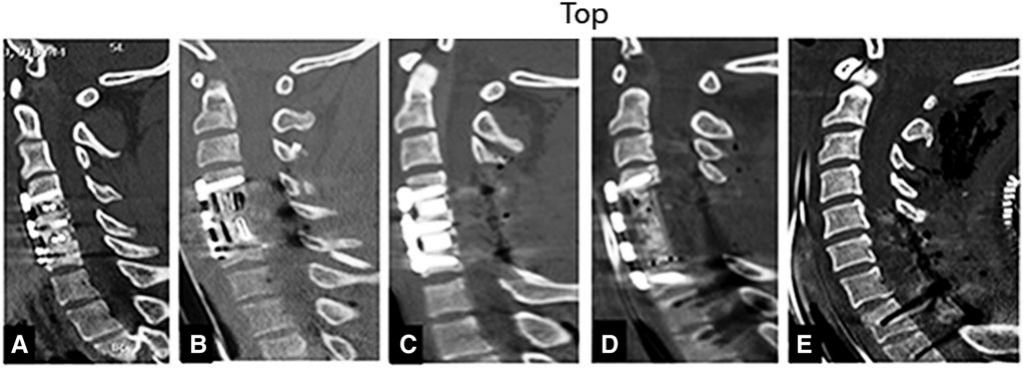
Midsagittal CT scan of cervical spine indicating (A) anterior cervical discectomy and fusion (ACDF); (B) anterior cervical corpectomy and fusion (ACCF); (C) Combined ACDF and three level laminectomy and posterior spinal fusion (PSF); (D) ACCF (two levels) and Laminectomy (two levels) and PSF; and (E) three levels of laminectomy and PSF.
